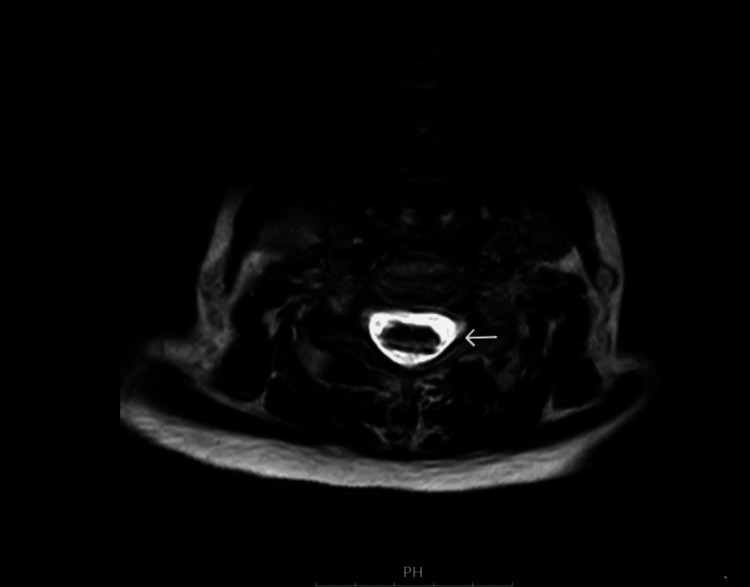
Axial view of the T2 sequence of MRI spine shows hyperintensity
